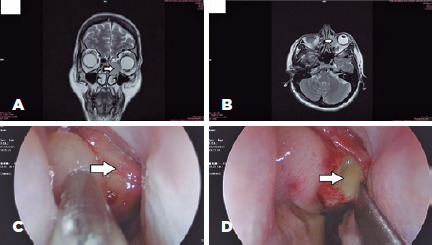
Magnetic resonance imaging and endoscopic findings. Magnetic resonance
